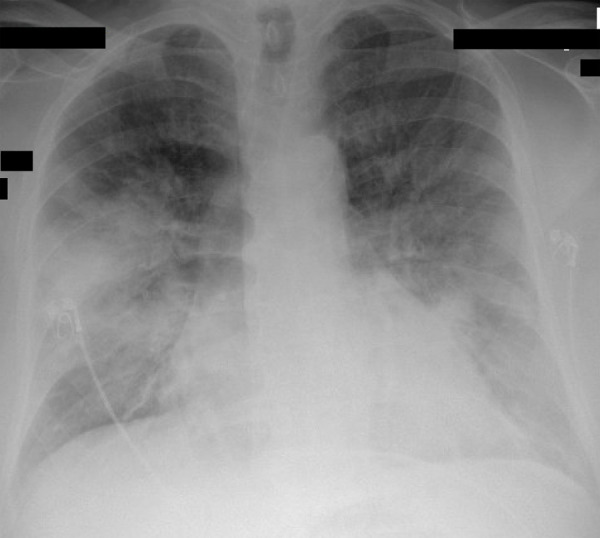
Chest X-ray at day of admission showing diffuse bilateral infiltrates greater on the right than left.
